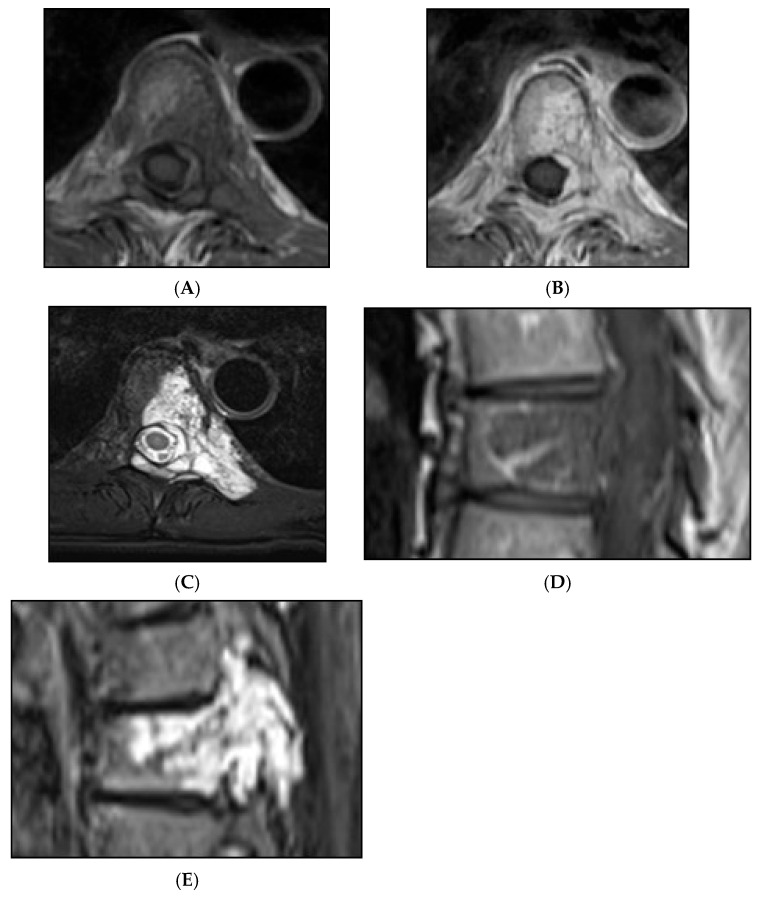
Aggressive spine haemangioma with involvement of the vertebral body and posterior elements with cortical expansion. (A) Axial T1W MRI. (B) Axial T1W post-contrast MRI. (C) Axial STIR MRI. (D) Sagittal T1W MRI. (E) Sagittal STIR MRI.
